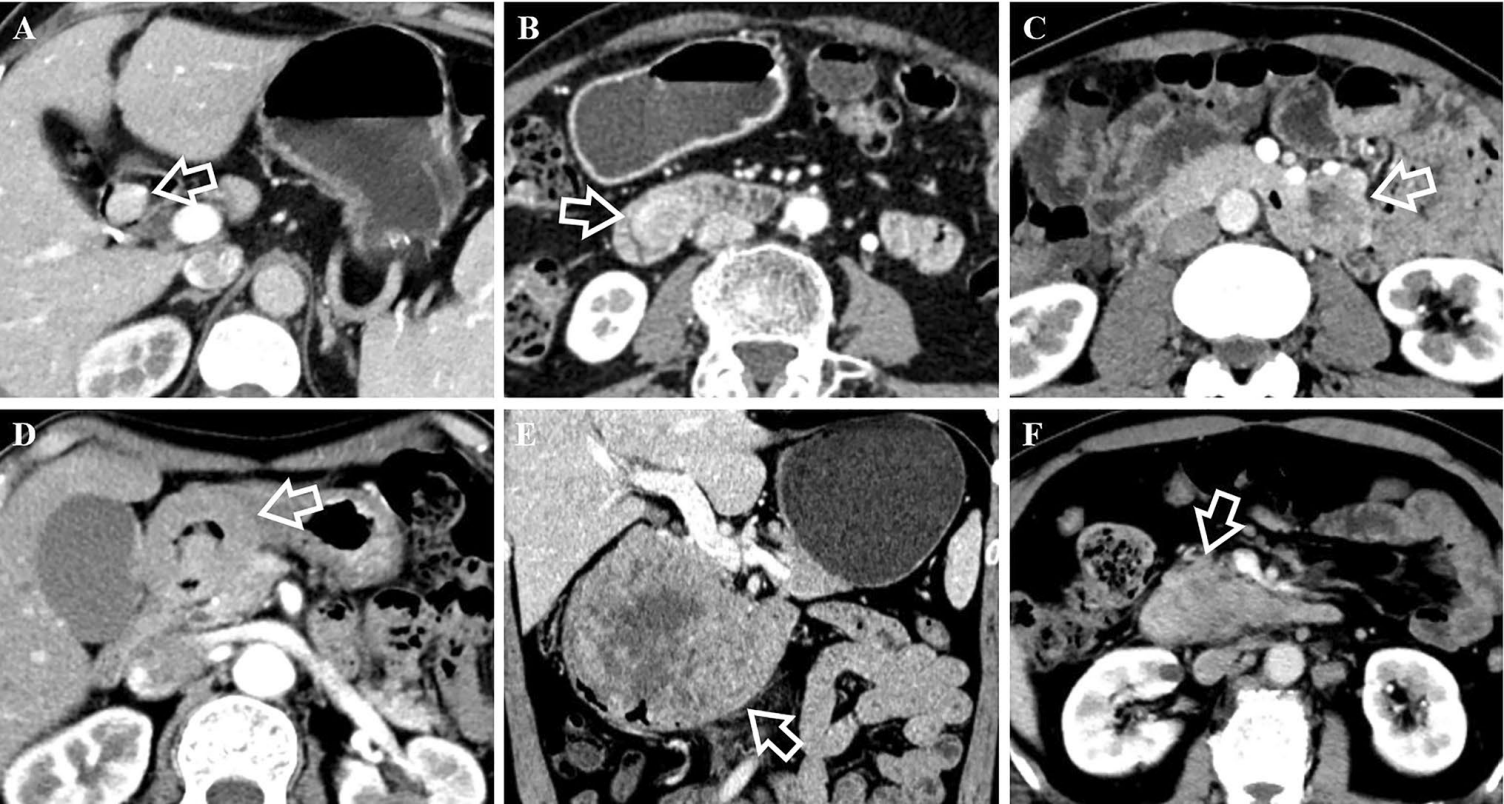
Portal venous phase enhanced CT scans of six examples of dNENs. A 60-year-old female with a G1 dNEN in the bulb part of the duodenum (white arrow). CT attenuation value of the tumor is 134.25 HU. Tumor recurrence was not noted during the 22-month follow-up period. B 74-year-old female with a G1 dNEN in the ampullary area (white arrow). CT attenuation value of the tumor is 129.16 HU. Tumor recurrence was not noted during the 10-month follow-up period. C 60-year-old male with a G2 dNEN in the ascending part of the duodenum (white arrow). CT attenuation value of the tumor is 128.96 HU. Tumor recurrence was not noted during the 11-month follow-up period. D 77-year-old male with a duodenal NEC presenting as wall thickening in the bulb part of the duodenum (white arrow). CT attenuation value of the tumor is 84.39 HU. The patient deceased at 5 months after palliative gastrojejunostomy. E 46-year-old male with a large G3 dNEN in the descending part of the duodenum (white arrow). The solid and cystic lesion has superficial ulceration but no obstructive biliary and/or pancreatic duct dilation. CT attenuation value of the tumor is 76.57 HU. The patient had synchronous liver metastases and was still alive during the 18-month follow-up period, but liver metastases continued to progress. F 65-year-old male with a duodenal NEC in the ampullary area (white arrow). The ill-defined lesion has obstructive biliary dilation. CT attenuation value of the tumor is 77.49 HU. The patient deceased at 6 months after endoscopic biopsy
